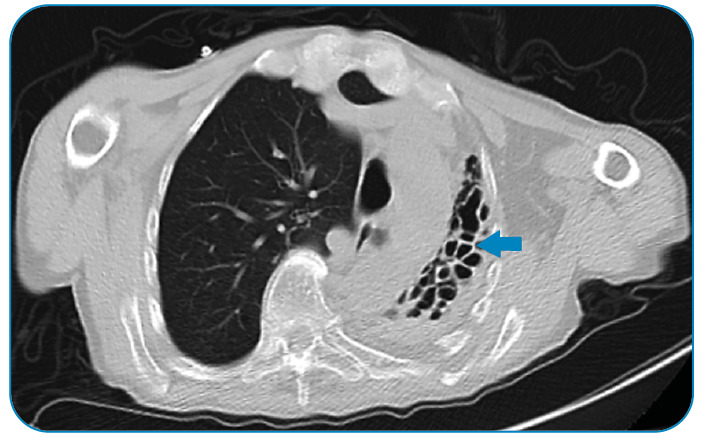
Thorax computed tomography showing unilateral tuberculous lung destruction (arrow).
